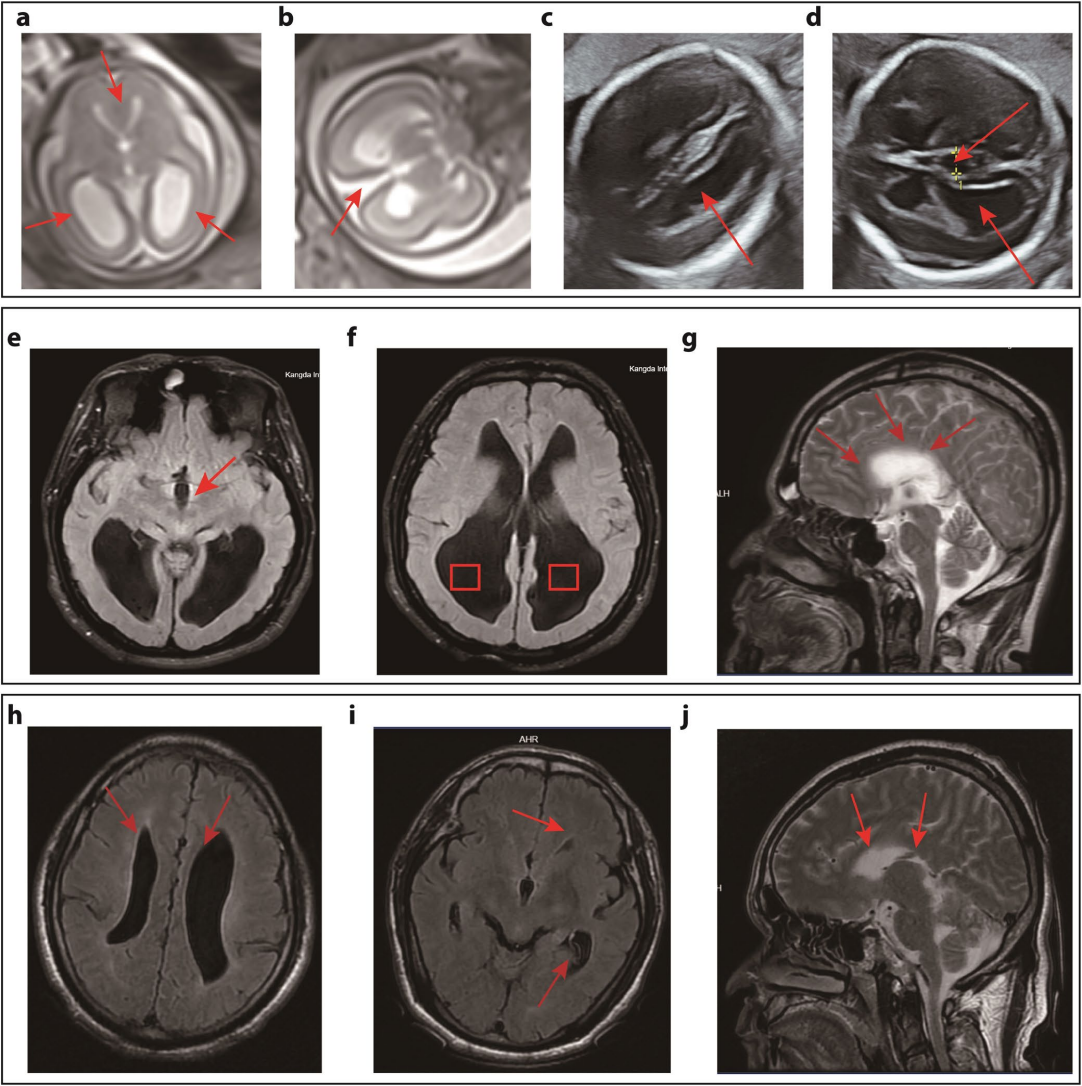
Neuroimaging of the proband and affected relatives. (a–d) Neuroimaging of the male proband at 23 weeks gestation. Fetal MRI (a, b) and ultrasound (c, d) show key features of *L1CAM*‐associated hydrocephalus, including agenesis of the corpus callosum and severe ventriculomegaly. (e–j) Brain MRIs of two affected maternal uncles (Uncle 1: e–g; Uncle 2: h–j) display characteristic findings, such as an absent corpus callosum and enlarged, malformed ventricles.
